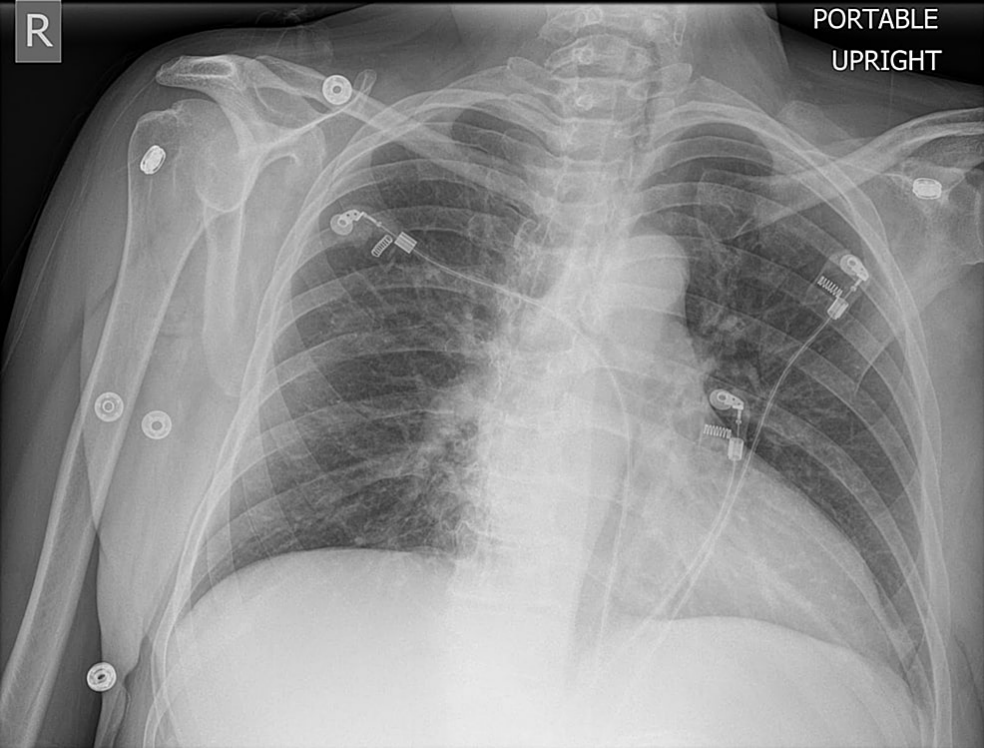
CXR negative for any acute cardiopulmonary process. CXR: chest x-ray.
